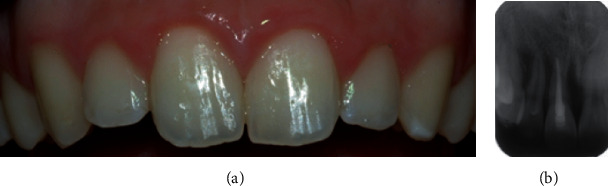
(a) Clinical and (b) radiographic view of the upper incisor at the 3-year follow-up visit.
